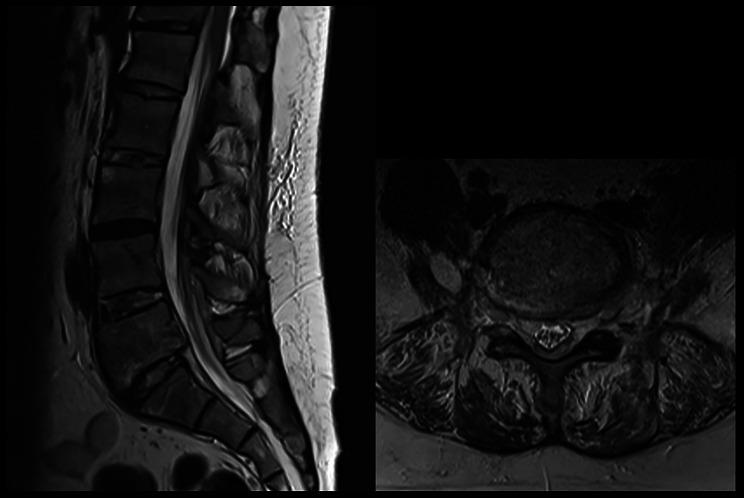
T2-weighted magnetic resonance (MR) images taken six days after injury show spontaneous regression of hematoma
